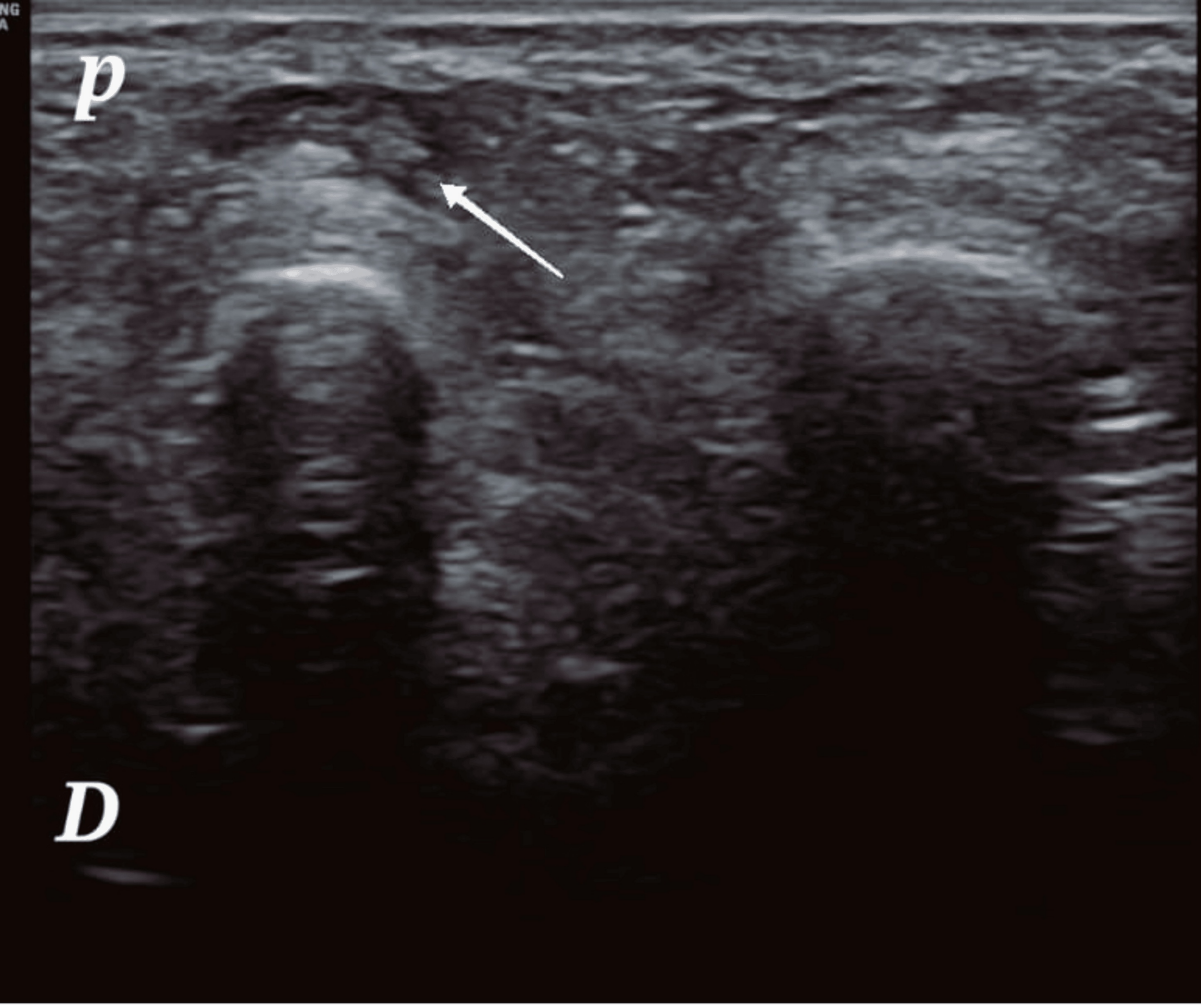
Ultrasound image of a Morton’s neuroma. p – Indicates the plantar aspect of the foot. D – Indicates the dorsal aspect of the foot.
